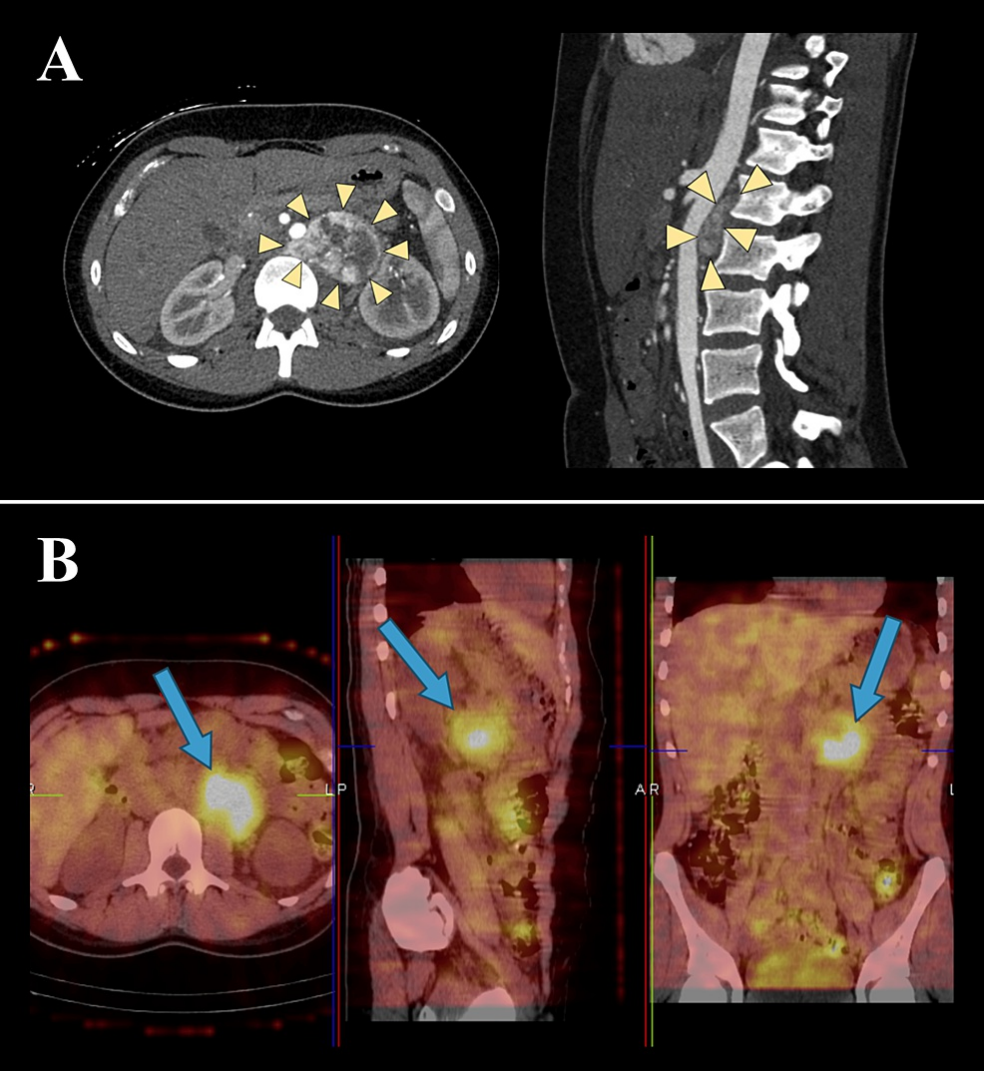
Patient 1 - contrast-enhanced CT scan of the abdomen (A) and (B) SPECT/CT scan with iodine-123 MIBG Yellow arrowheads frame the margins of the mass and blue arrows point to the diffuse activity accumulation in the mass area, separate from the left adrenal. CT, computed tomography; MIBG, meta-iodobenzylguanidine; SPECT, single-photon emission computed tomography
